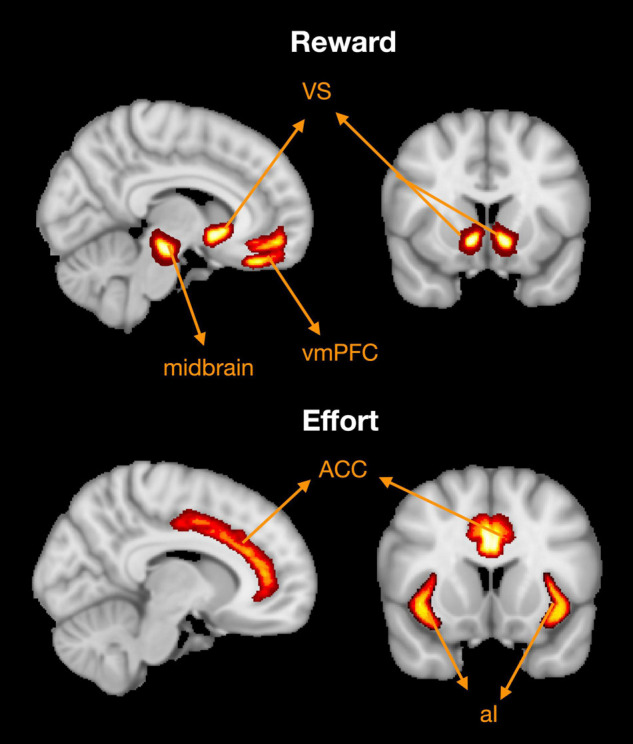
Brain regions associated with reward and effort processing. Based on a meta-analysis of functional MRI studies (Pessiglione et al., 2018). Reward processing associated with activation in the ventromedial pre-frontal cortex (vmPFC), ventral striatum (VS), and ventral tegmental area within the midbrain. Effort processing clusters are principally found in the dorsal anterior cingulate cortex (ACC) and anterior insula (AI). Adapted from Figure 5 in Pessiglione et al. (2018).
